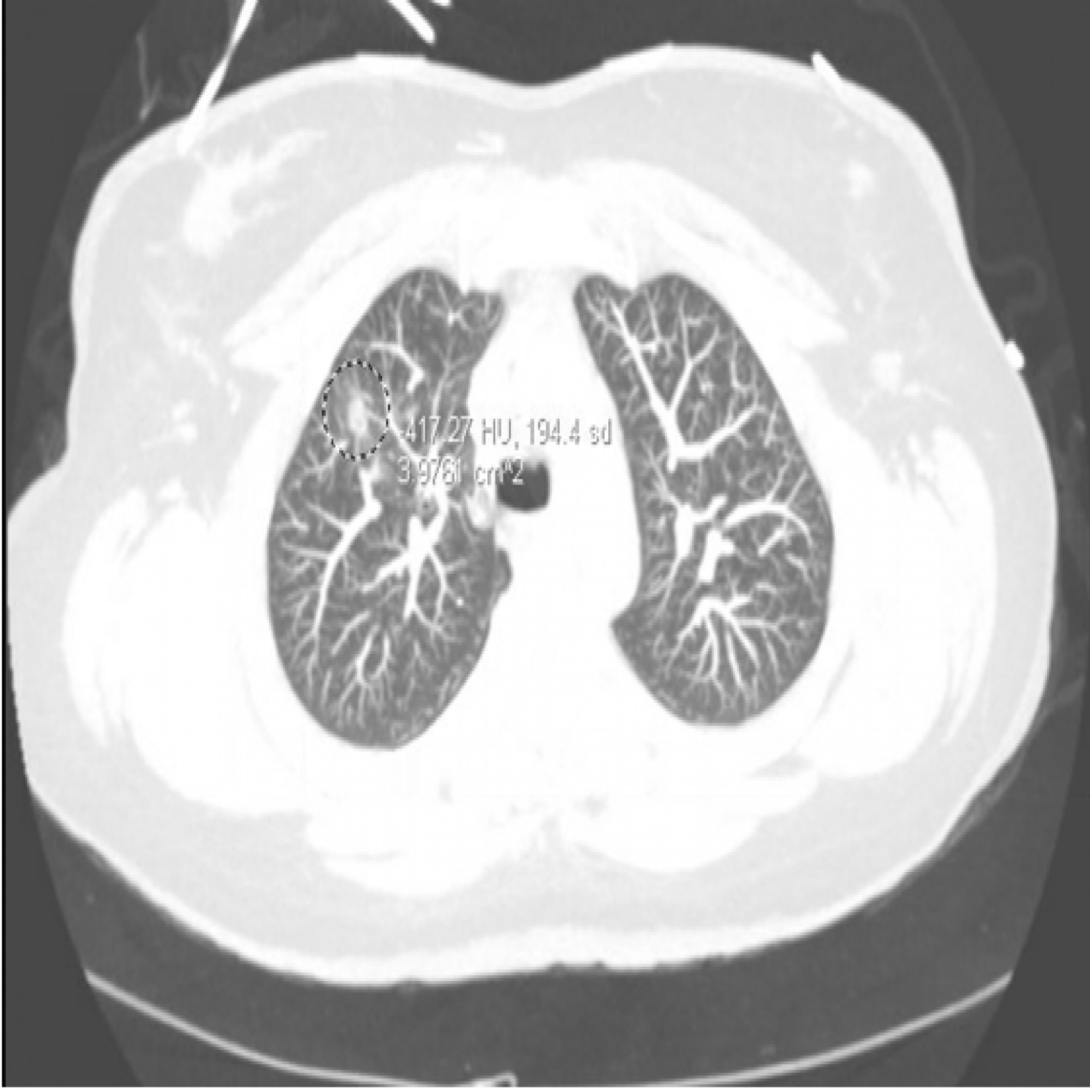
Computed tomography (CT) of the chest in axial view revealing the small focus of ground-glass opacity and its subcentimeter parenchymal cysts within the right upper lobe (encircled).
